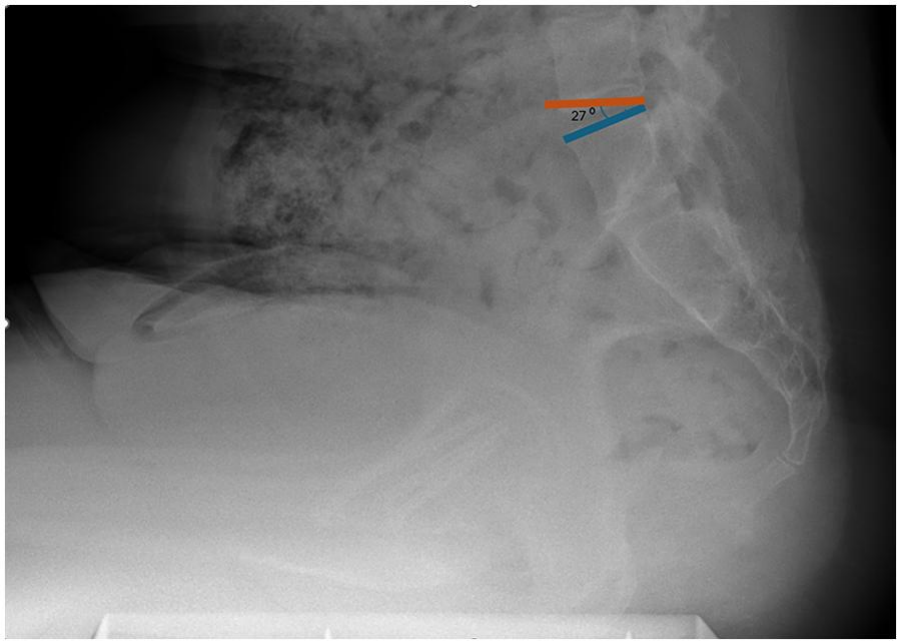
Measurement of sacral slope (SS). The angle between the horizontal reference line and the superior endplate of S1 is identified on the lateral lumbosacral radiograph.
